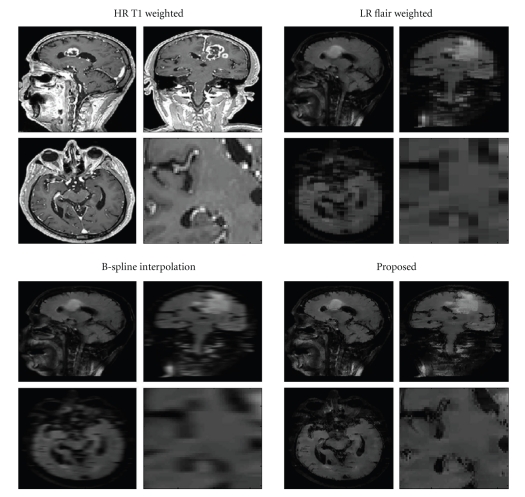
Comparison of real clinical data experiment. Top-Left: HR T1 data. Top-Right: LR FLAIR volume. Bottom-left: B-Spline reconstruction. Bottom-right: reconstruction using the proposed method. In each case a close-up of the coronal slice is presented to better show how the proposed method provides a less blurry reconstruction.
